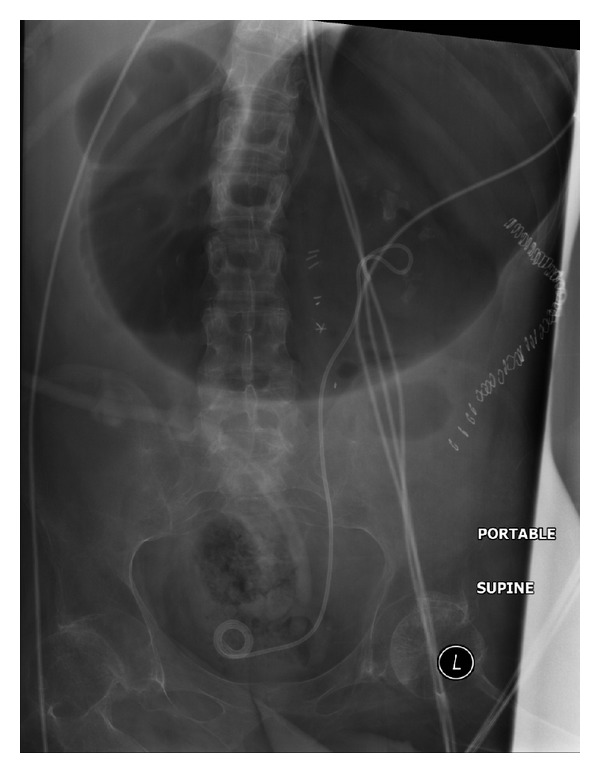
X-ray of abdomen, taken on 11 August 2009, revealed gastric distension.
